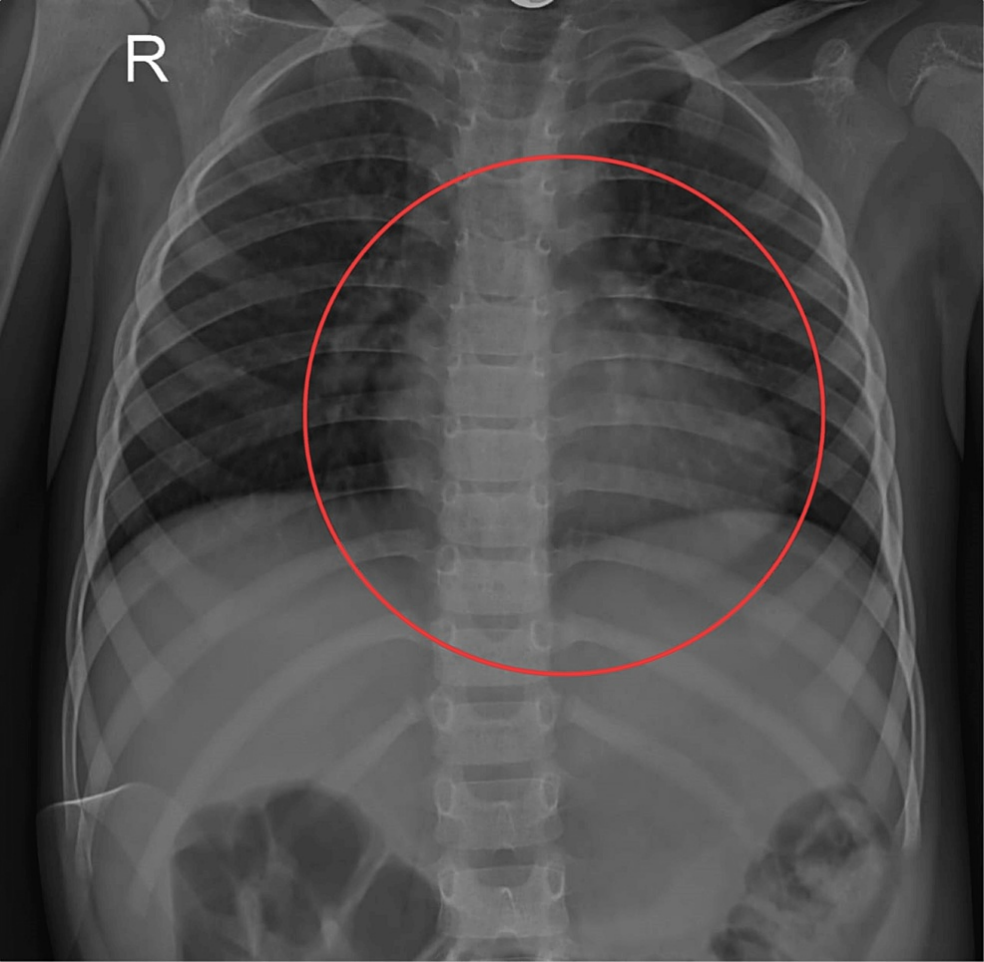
Preoperative chest radiograph: posteroanterior view The circle represents a boot-shaped heart with hyperlucent lungs
